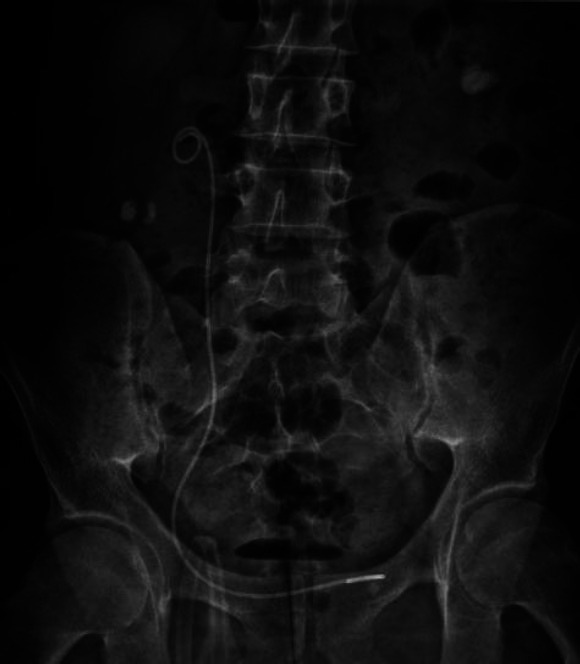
KUB radiography of Patient 2 on the postoperative 3rd day, after Re-Double-J stent insertion.
